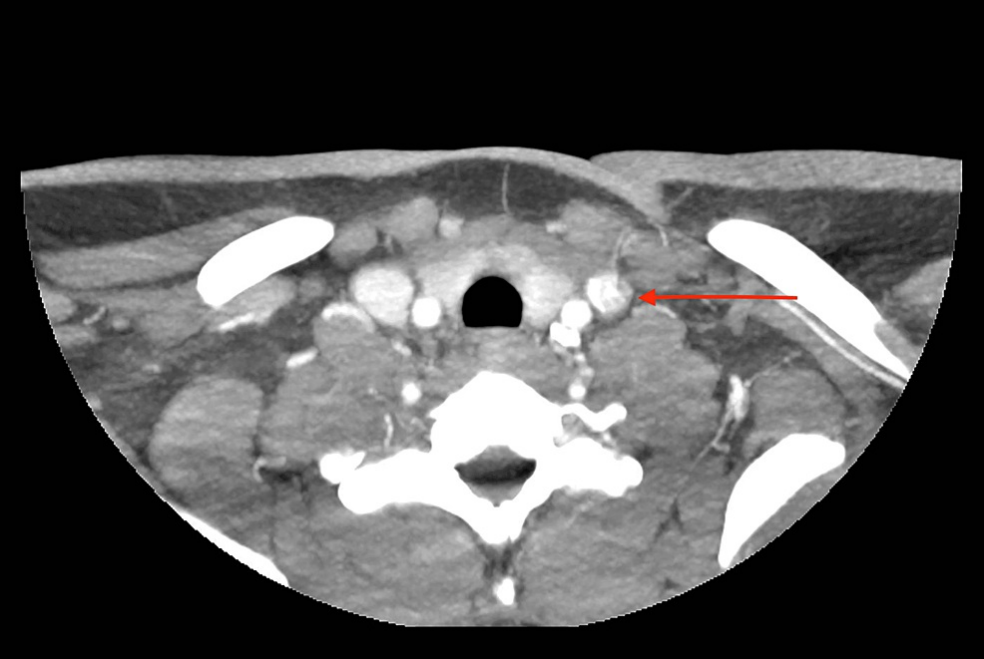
Arrow showing an ill-defined low-attenuation in the left internal jugular vein on CT angiogram of the head and neck.
